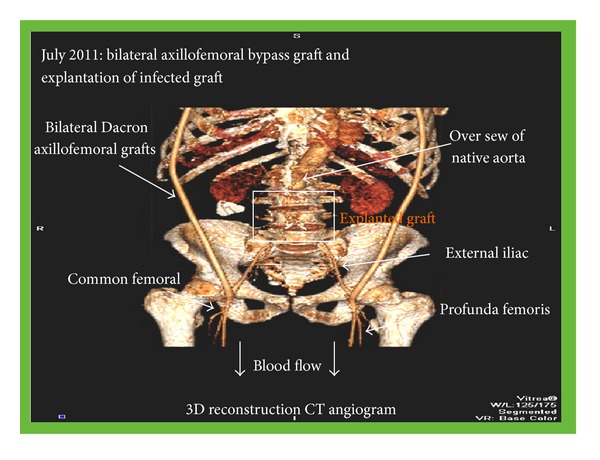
3D-CT angiogram demonstrating removal of the infected aortic graft and extra-anatomical bypass.
